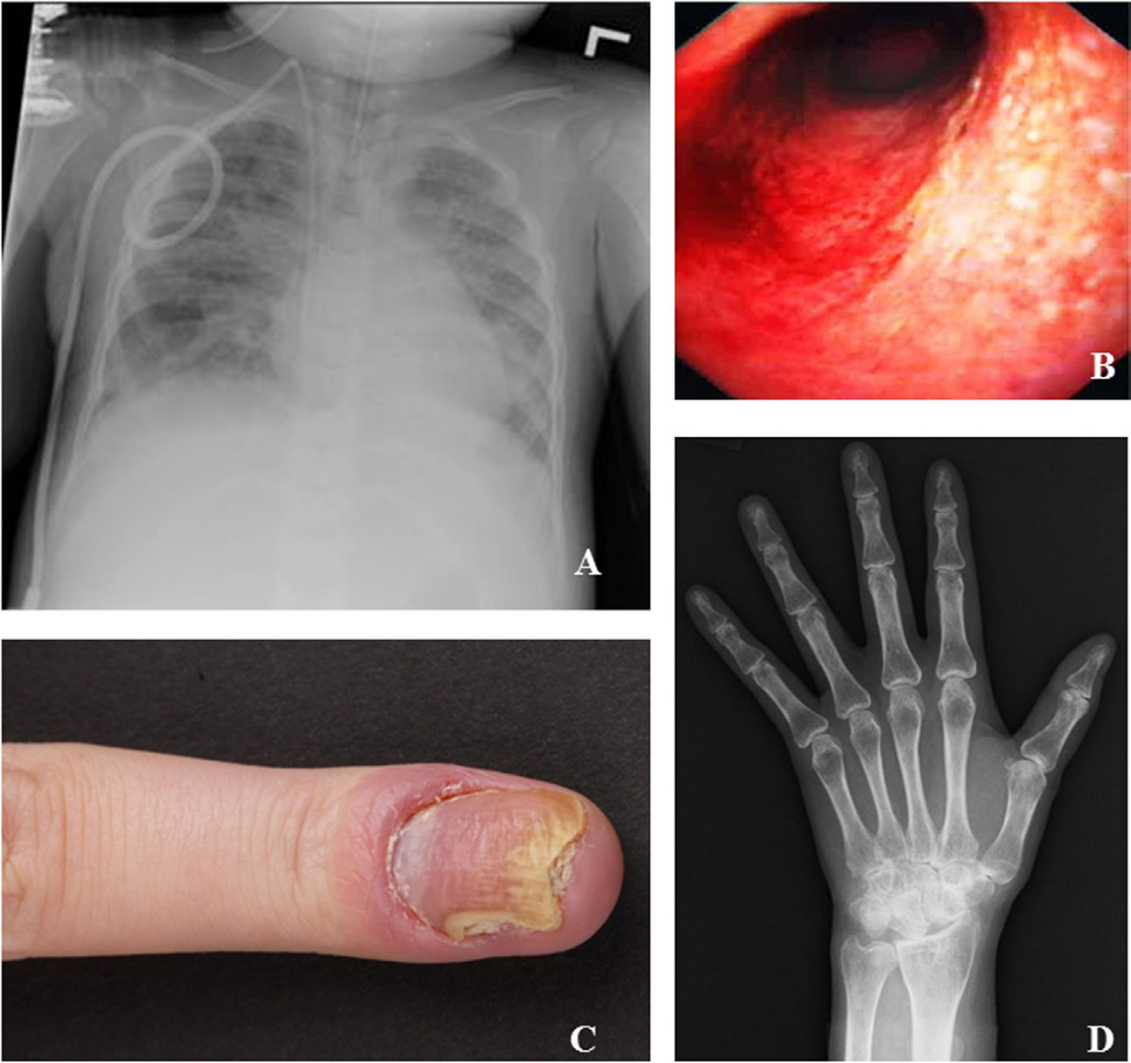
Specific clinical manifestations of inducible co-stimulator (ICOS) deficiency. (A) Chest X-ray of patient #12 at the time of respiratory failure shows multifocal patchy consolidative opacities with moderate pulmonary edema. (B) Colonoscopy of patient #9 shows inflammatory bowel disease. (C) Finger of patient #7 with psoriasis of the nail and secondary *Candida* infection. (D) Hand X-ray of patient #10 shows erosive changes suspicious of rheumatoid arthritis.
